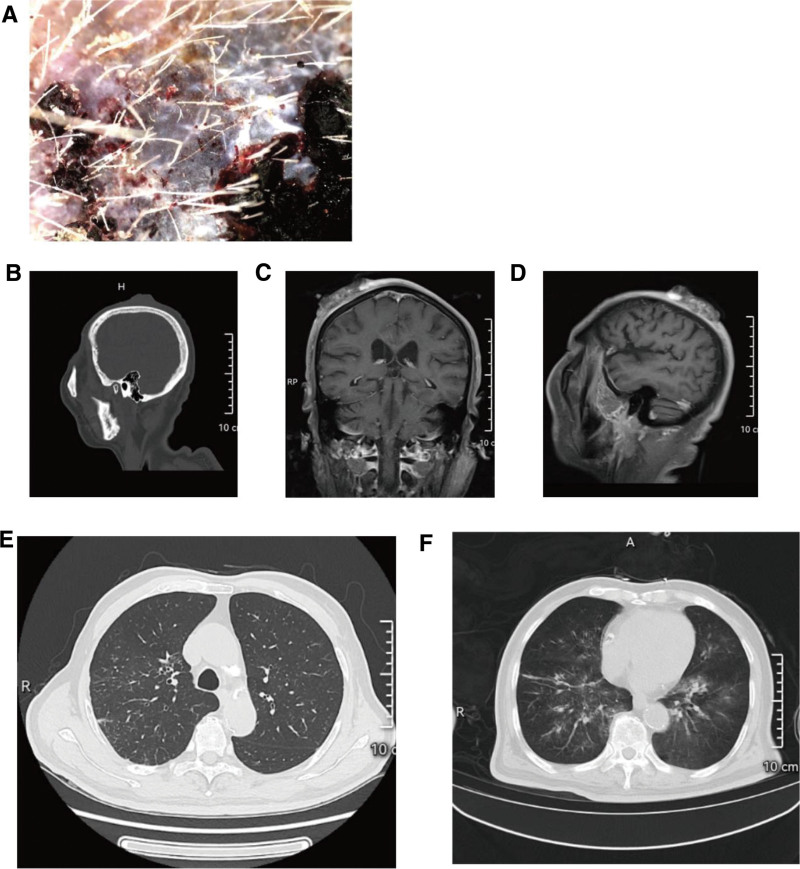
(A) Dermoscopic findings of the scalp mass of the patient on admission. (B) The patient’s head CT (sagittal view) at admission. (C) The patient’s head MRI (coronal view) at admission. (D) The patient’s head MRI (sagittal view) at admission. (E) Chest CT findings of the patient on admission. (F) Chest CT findings of the patient at the time of sudden massive hemoptysis, 5 months after surgery. CT = computed tomography, MRI = magnetic resonance imaging.
